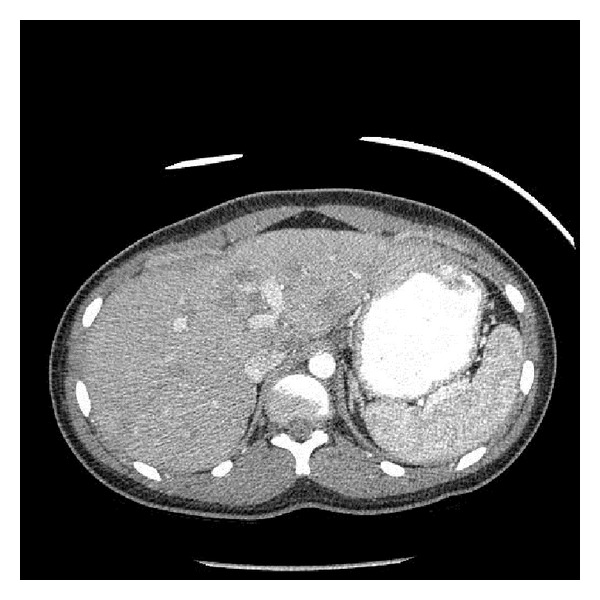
Abdominal CT showing multiple poorly defined low attenuation lesions.
